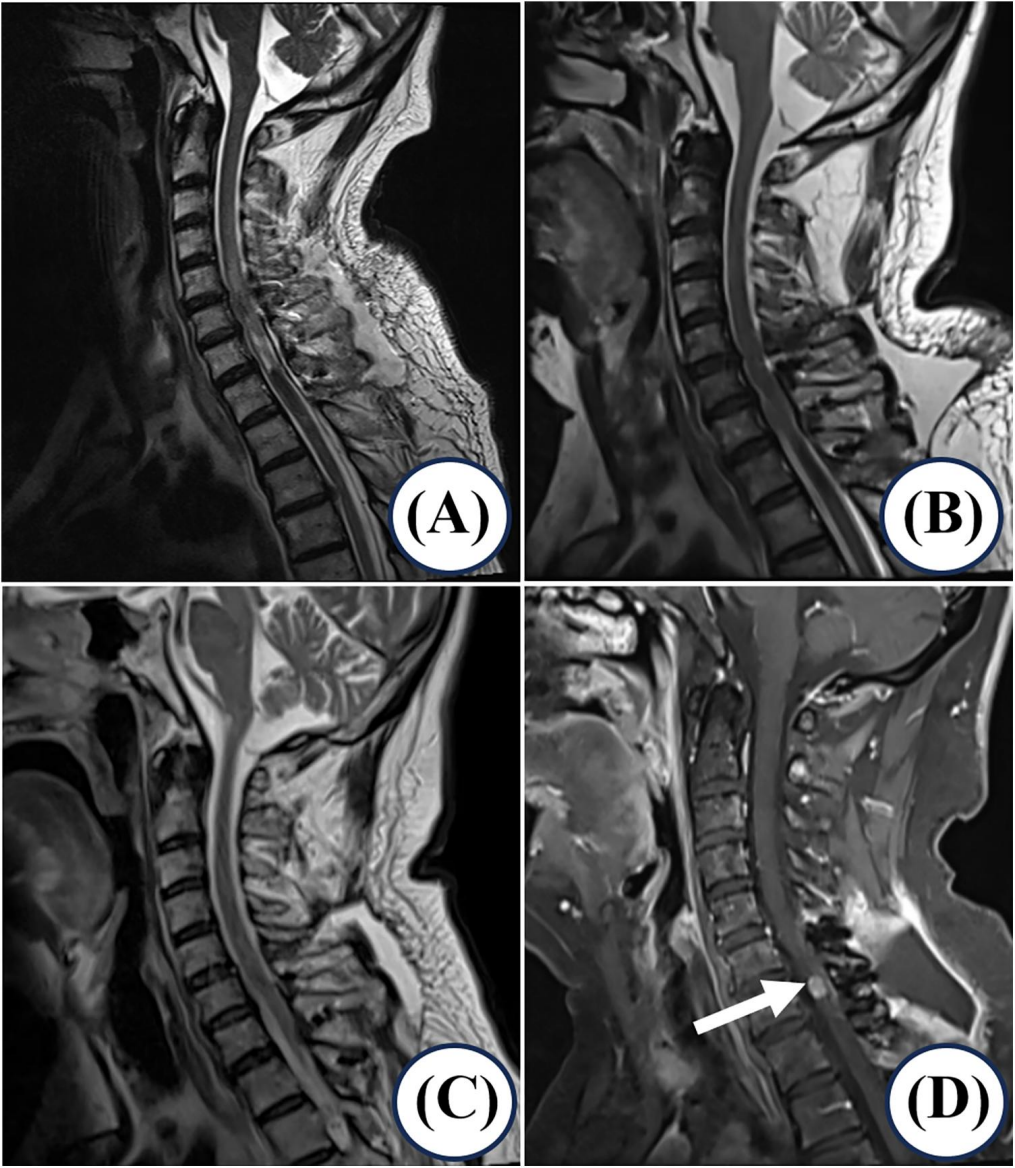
Postoperative imaging follow-up demonstrating treatment response and late recurrence. (A) Immediate postoperative MRI demonstrates expected postoperative changes, with a small posterior epidural fluid collection at the surgical site, consistent with a minor cerebrospinal fluid (CSF) leak. (B) The 3-month follow-up cervical MRI shows an interval increase in the volume of subcutaneous and epidural fluid along the posterior surgical tract from C5 to T1, indicating a persistent CSF leak. (C) A 6-month follow-up cervical MRI shows partial resolution of the previously noted subcutaneous edema and cystic formation. (D) At the 11-month follow-up, contrast-enhanced MRI identifies a 7-mm ring-enhancing nodular lesion (arrow) with T2-weighted fat-suppressed hyperintensity at the C7 level, resulting in mild spinal cord compression.
